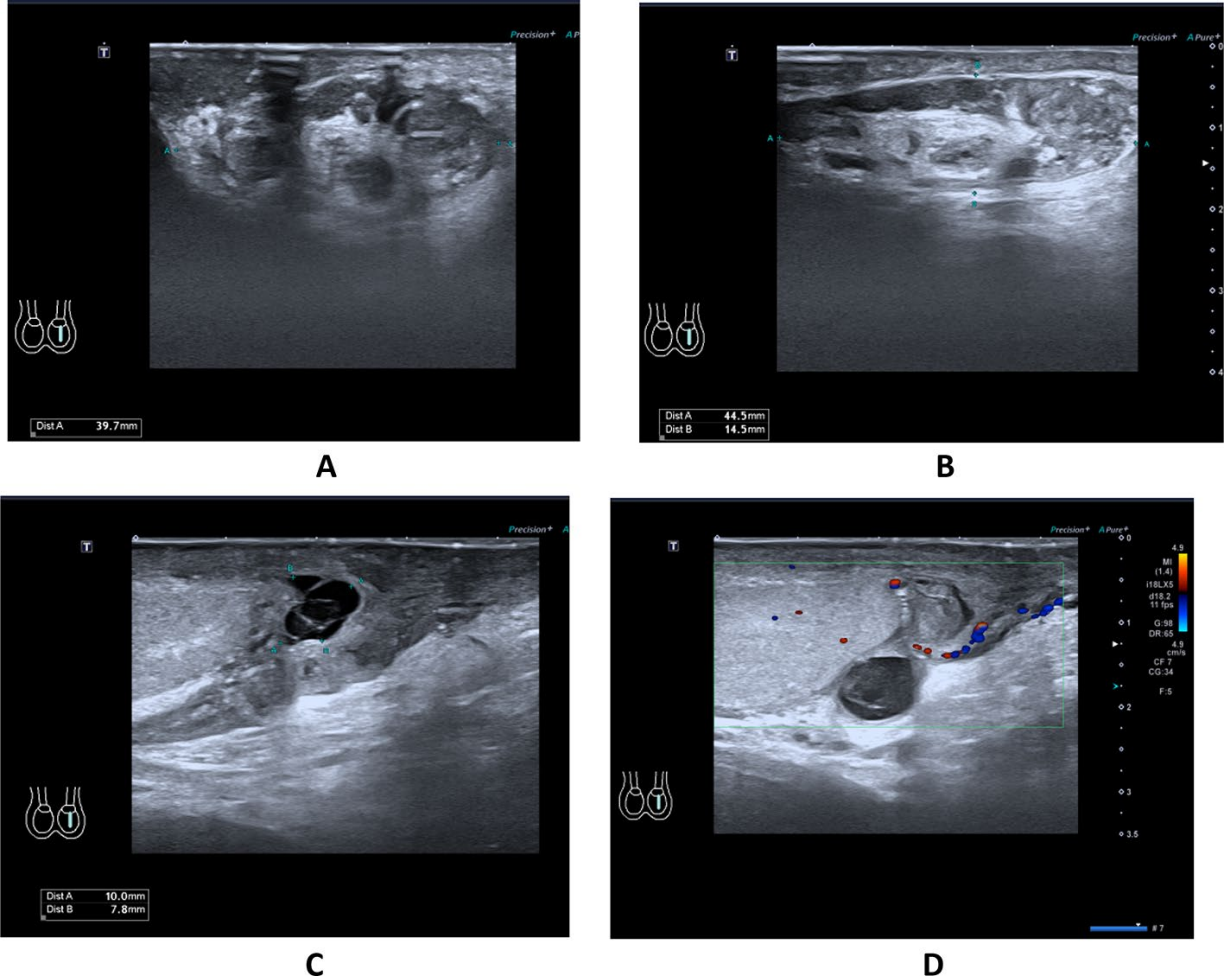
Follow-up ultrasound examination; The surgical site demonstrated disorganized soft tissue structures exhibiting heterogeneous echogenicity, measuring approximately 4.45 × 1.45 × 3.97 cm (A, B). Multiple anechoic areas were identified, one of which measured approximately 1.0 × 0.78 cm and exhibited poor internal echogenicity with visible septations (C, D)
